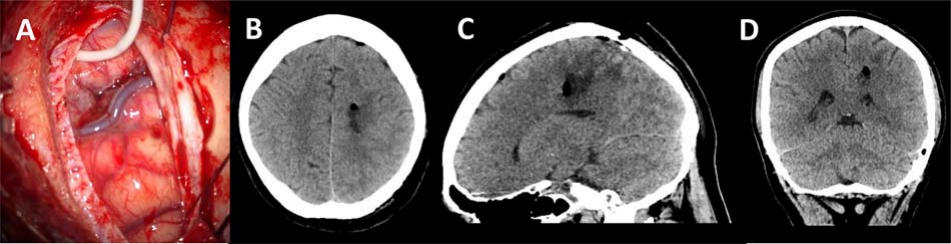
Post-operative imaging; (A) post-decannulation brain surface after transsulcal approach; (B–D) axial, sagittal and coronal CT images showing collapse of the surgical tract and no overt complications.
